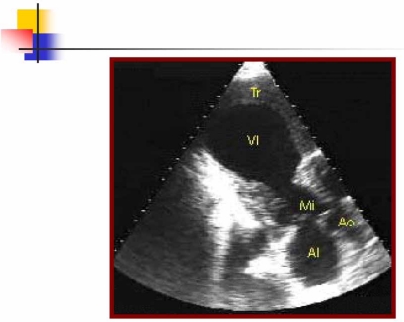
Transthoracic echocardiography shows a left ventricular aneurysm (VI) in a patient with history of acute myocardial infarction.
